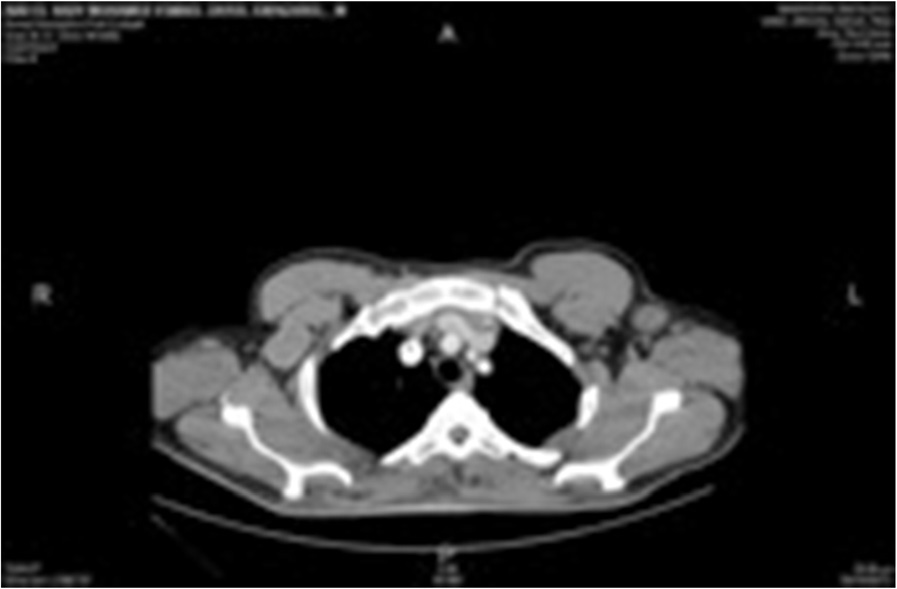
Computed tomography showing significant axillary lymph node
